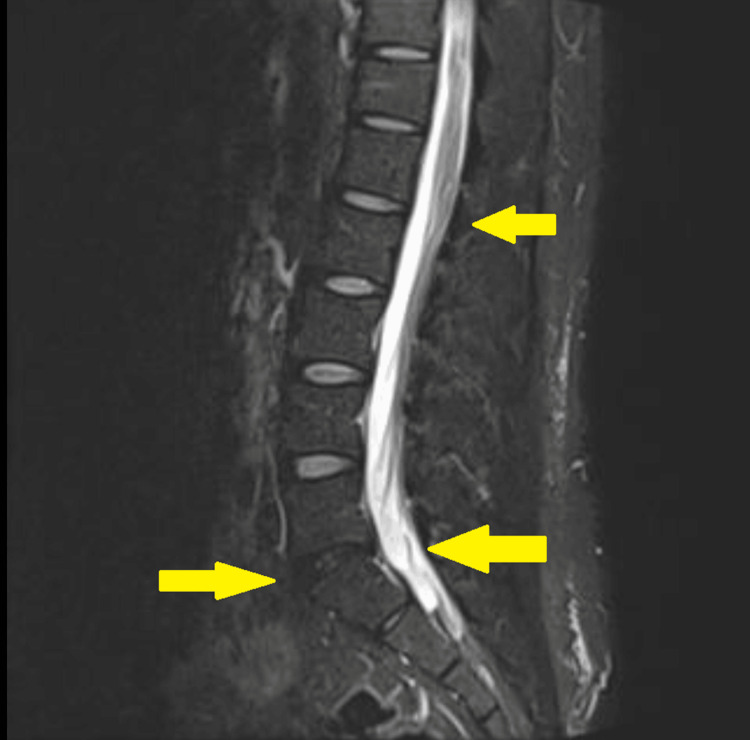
Normal MRI of the lumbosacral spine Sagittal T2-weighted magnetic resonance imaging (MRI) of the lumbosacral spine. The yellow arrows indicate normal anatomical structures, including the spinal canal, cauda equina, and intervertebral discs, with no evidence of compressive or inflammatory neuraxial pathology. This normal finding was crucial as a diagnosis of exclusion to rule out structural causes for the patient's symptoms, thereby supporting the clinical diagnosis of Complex Regional Pain Syndrome (CRPS).
